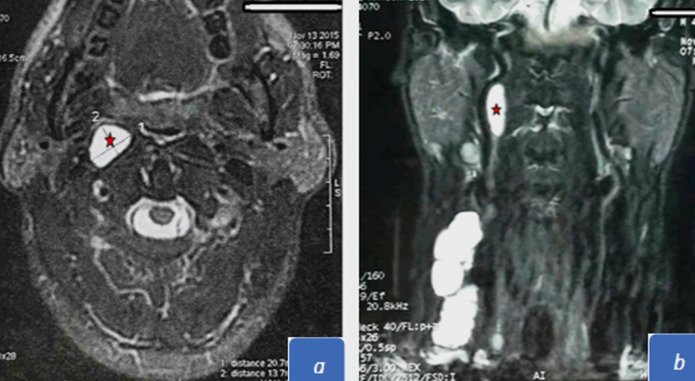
MRI showing enhanced lymph node swelling in the right retropharyngeal space which doesn´t dislocate the intenal carotid artery; a) axial T2 weighted with fat-suppressed; b) coronal T2 weighted with fat-suppressed
